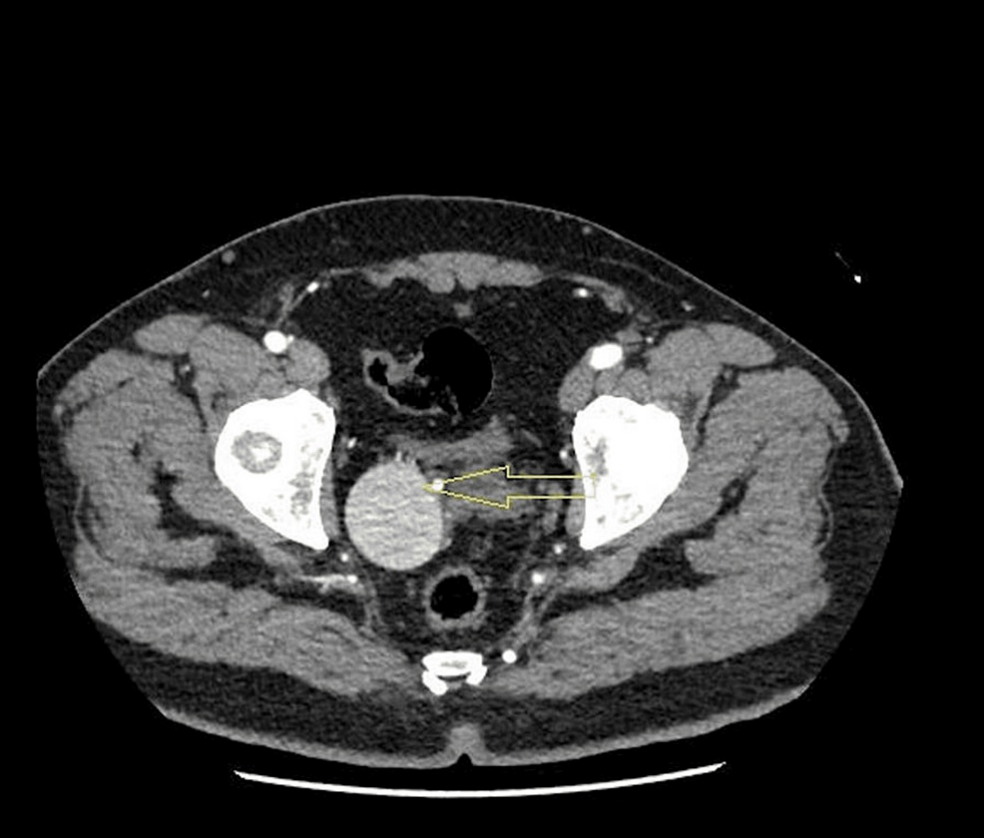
CT angiography The yellow arrow shows a pear-shaped aneurysm measuring 6.5 x 3.5 cm at the right-side pelvic cavity. The aneurysm can be seen displacing the adjacent urinary bladder seminal vesicles and prostate CT: computed tomography
